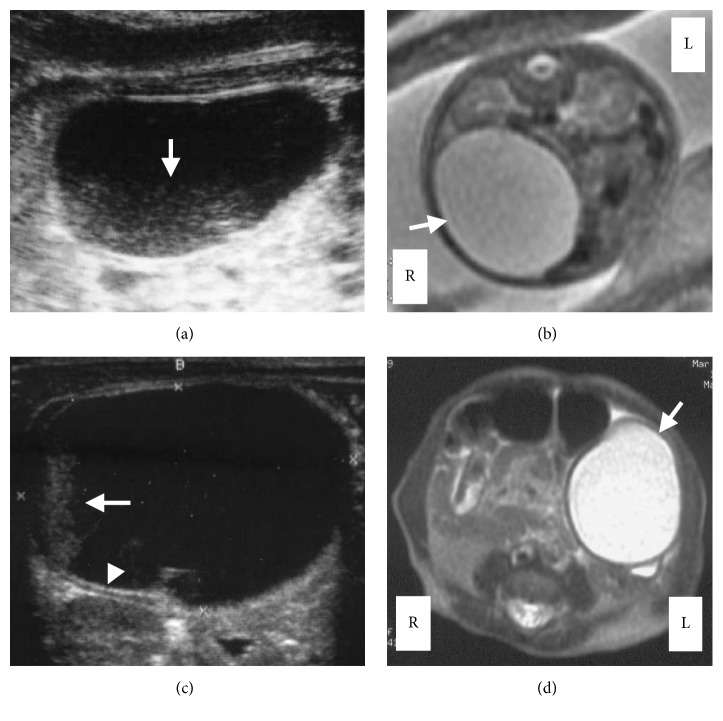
MR and US images of the abdominal mass. (a) Fetal ultrasonogram at 37 weeks' gestation: longitudinal section through the abdomen of the fetus shows a 4 × 3 cm cystic mass with sedimented echoes (arrow). (b) Fetal MR image at 28 weeks' gestation: axial T2-weighted image shows a cystic mass lesion in the right side of the fetal abdomen (arrow). (c) Postnatal longitudinal ultrasonogram on day 0 shows a 4.5 × 3.5 cm cystic mass with floating internal echoes in the right abdomen. Note the fluid-debris level (arrow) and muscular rim sign (arrowhead). (d) Axial T2-weighted MR image on postnatal day 16 reveals a hyperintense cyst in the left lower abdomen (arrow).
